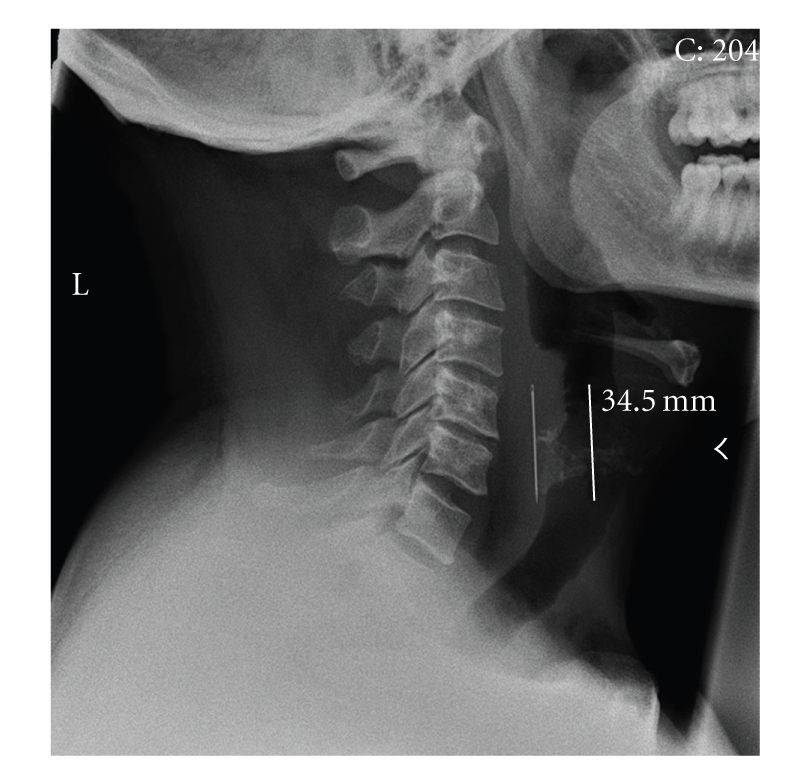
Lateral cervical spine radiograph.
